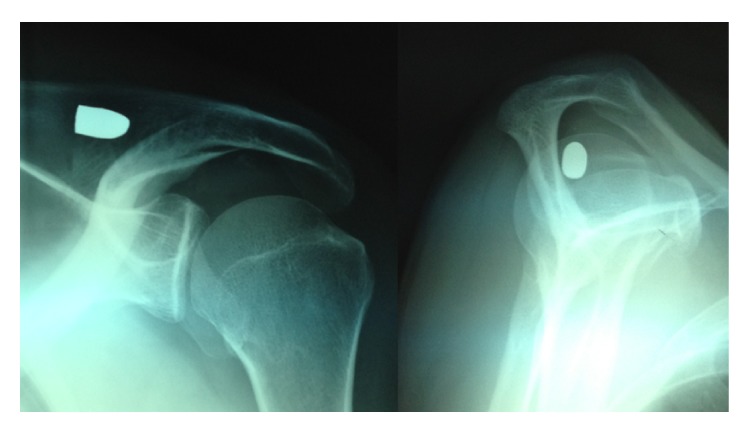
Preoperative anteroposterior and axial radiographs showing the bullet in the supraspinatus compartment of the shoulder.
